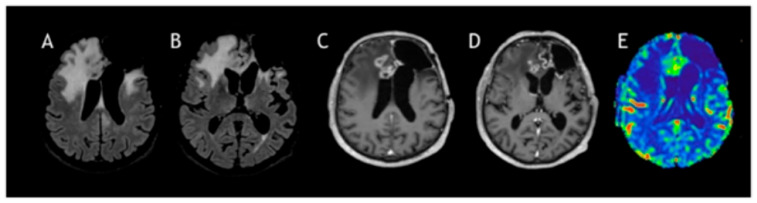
Progression of glioblastoma, using techniques MRI FLAIR (A,B), post-contrast T1-weighted images (C,D), and CBV map (E). Follow-up studies show tumor progression with increased infiltration of the corpus callosum and bilateral frontomesial regions, associated with mass effect, perilesional edema, irregular contrast enhancement, and elevated CBV.
